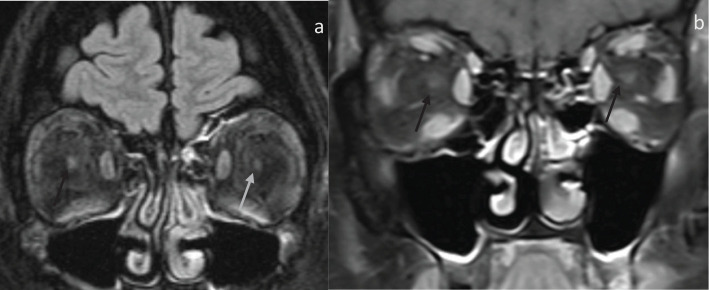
53-year-old presenting with sequential horizontal field vision loss with fundoscopic exam demonstrating bilateral grade 4 optic disc edema. Clinical diagnosis was consistent with NAION. (A) Coronal fat-saturated FLAIR demonstrates FLAIR hyperintensity and indistinctness of the right intraorbital optic nerve (black arrow) compared to the left optic nerve (gray arrow). (B) Coronal fat, saturated post-contrast, demonstrates no enhancement within both intraorbital optic nerves (arrows).
